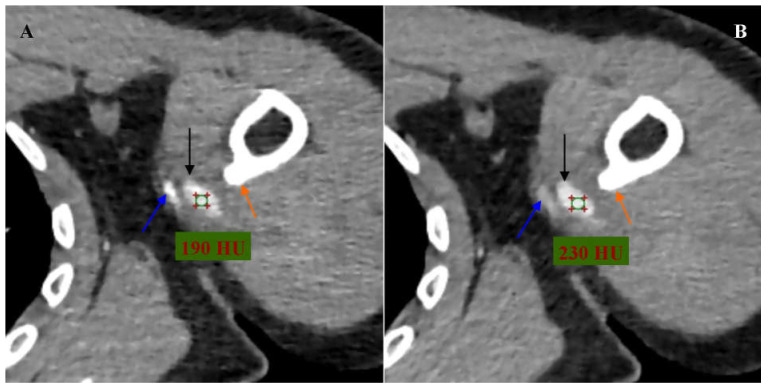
Computed tomography angiography of the left upper limb arteries. (A) Angiographic phase. Multiplanar reconstruction (MPR). Axial view. (B) Delayed phase. MPR reconstruction. Axial view. The blue arrow indicates the left brachial artery. The black arrow indicates a pseudoaneurysm of the left brachial artery. The orange arrow indicates the osteochondral exostosis of the left humerus. A green circle with red markers for tissue density measurement was placed within the lumen of the left brachial artery pseudoaneurysm. The values indicate the measured lumen density of the pseudoaneurysm of the left brachial artery. The density of the pseudoaneurysm lumen was approximately 190 HU in the angiographic phase and approximately 230 HU in the delayed phase, which indicated active inflow and delayed outflow of contrasted blood into the lumen of the pseudoaneurysm.
